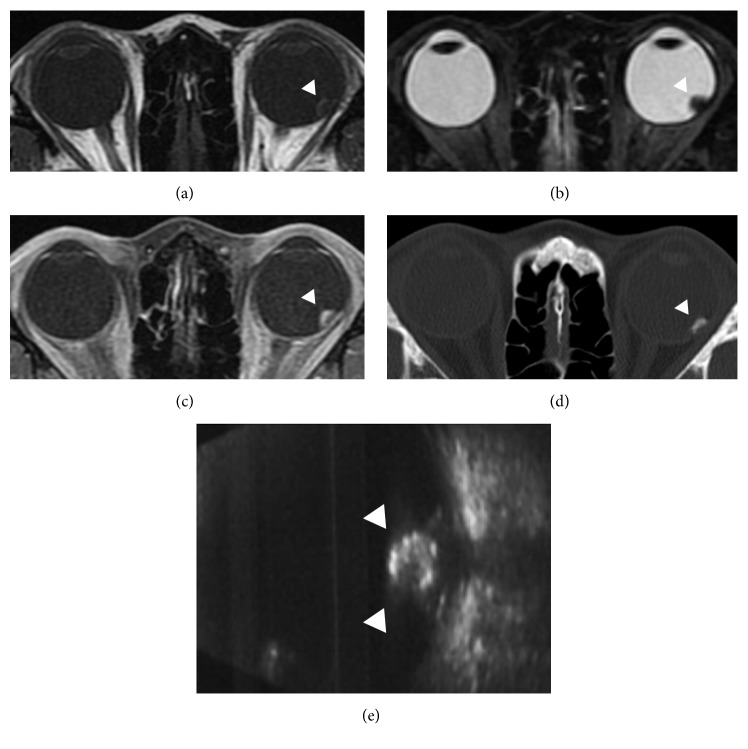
The tumor was depicted as high intensity on both T1-weighted (a) and gadolinium enhanced (c) MRI, low intensity on STIR MRI (b), and high density lesion on CT (d). The B-scan ultrasonography showed high internal reflectivity and acoustic shadowing (e) (arrow heads).
